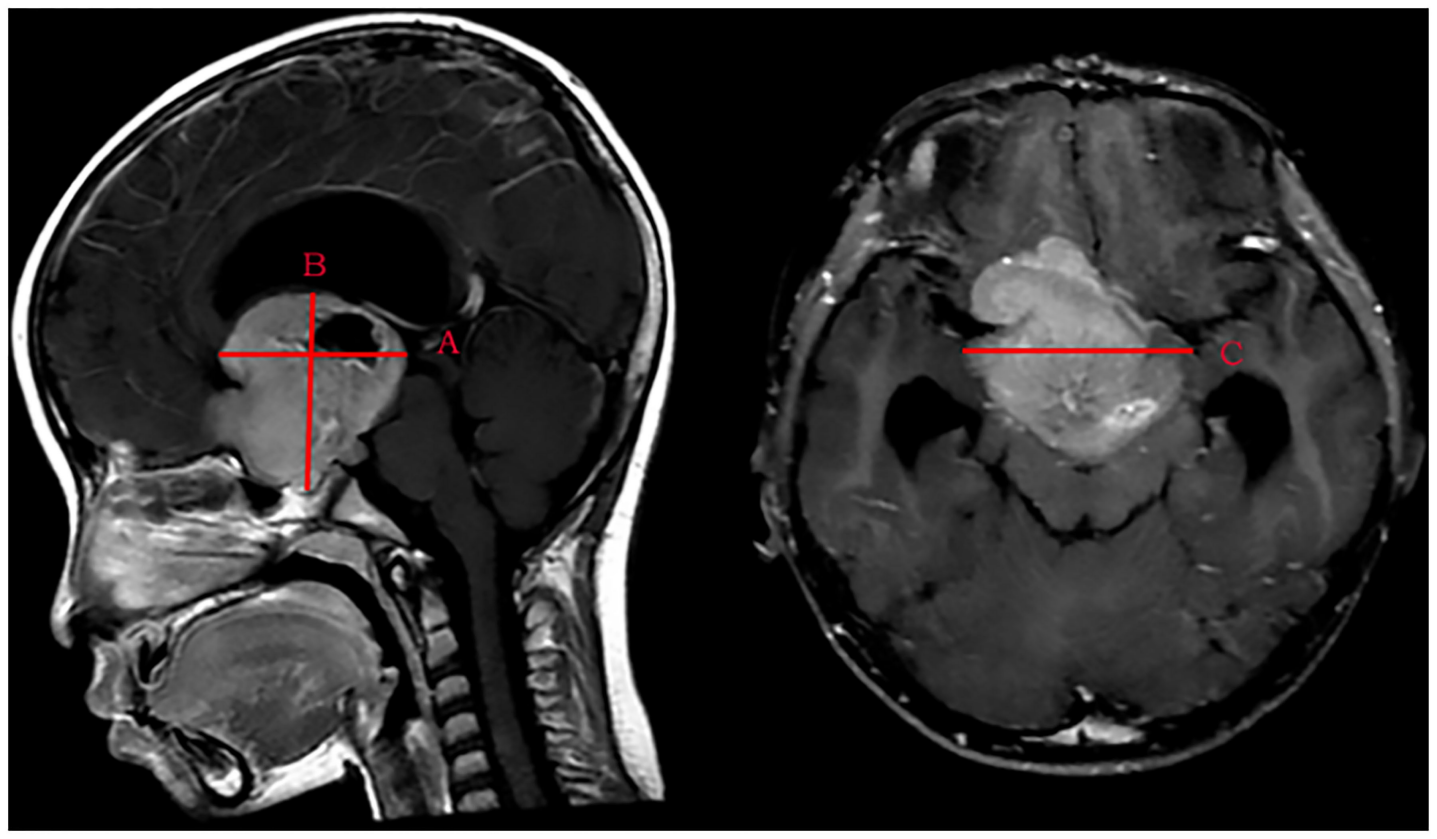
Measurement of the maximum distance on three standard planes of MRI images. (A) The maximum anteroposterior distance of the tumor is parallel to the AC-PC-line. (B) The maximum craniocaudal distance of the tumor is perpendicular to the axial plane. (C) The maximum transverse distance of the tumor is perpendicular to the sagittal plane.
